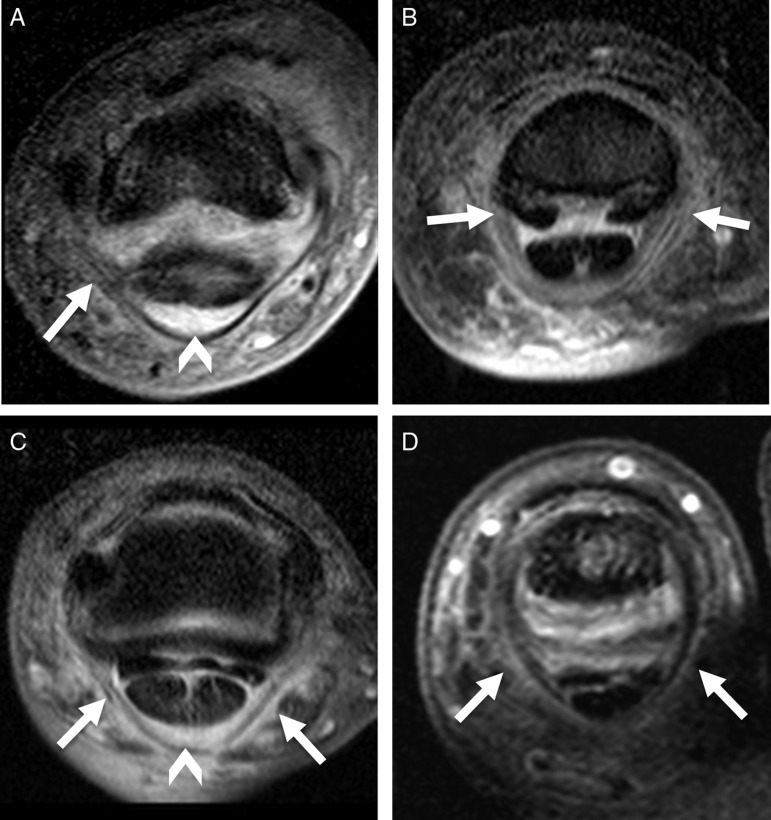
Finger pulleys and toe fibrous sheath abnormalities. Fat-saturated contrast-enhanced T1-weighted axial images. (A) Interphalangeal joint of the left thumb of a 57-year-old man. The A2 pulley region adjacent to the bone was unilaterally oedematous and ill-defined (white arrow). Area of enhanced high signal representing thickened flexor tenosynovium is also noted (arrowhead). (B) Distal site of proximal interphalangeal (PIP) joint of the left index finger of a 62-year-old man. There is oedematous change at bilateral A4 pulley attachments (white arrows). (C) PIP joint of the left index finger of same patient as (B). Symmetrical rim-like enhancement is observed around the A3 pulley (white arrows). The flexor tenosynovium is thickened as noted by the area of high signal (arrowhead). (D) PIP joint of the right 2nd toe of same patient as (2C) showing oedematous change of the fibrous sheath (white arrows).
